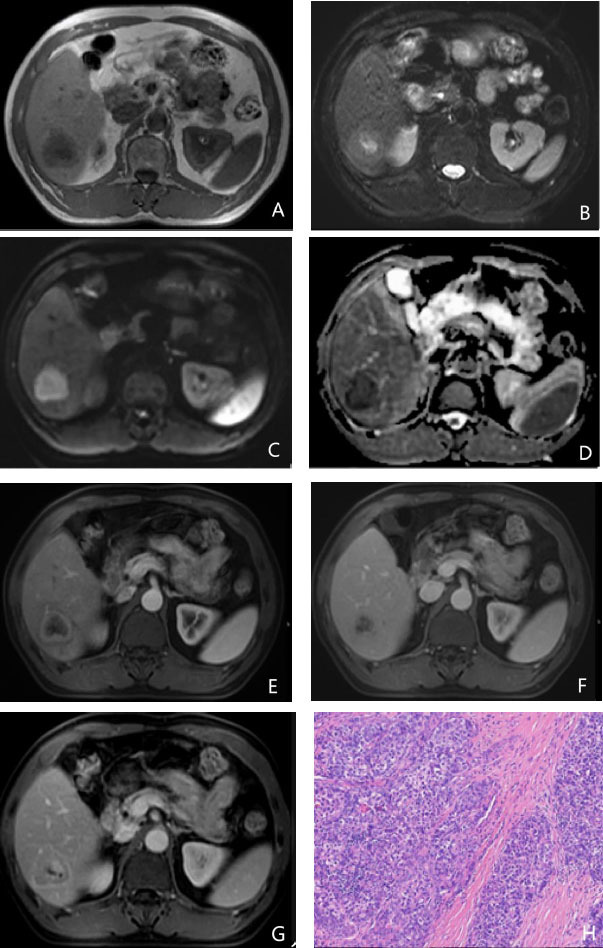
A 59-year-old male DPHCC patient. A: T1WI showed low signal. B: T2WI showed a heterogeneous, slightly high signal. C: DWI showed a high signal. D: ADC showed a low signal. E, F, G: After enhancement, the lesion showed ring-like high enhancement in the arterial phase and progressive enhancement in the portal venous and delayed phases. H: Pathological observation (10×) showed many fibrous tissues in the tumor.
